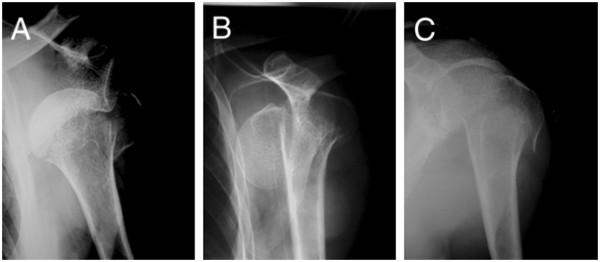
X-ray findings. Initial X-ray showed dislocation of the left shoulder, displaced fracture of the greater tuberosity and an undisplaced humeral anatomical neck fracture (A). After an initial trial for reduction by Stimson’s method, X-ray showed the complete displacement of the humeral head (B). After the fluoroscope-assisted closed reduction under general anesthesia, reduction was obtained with angulation between the humeral head and shaft (C).
